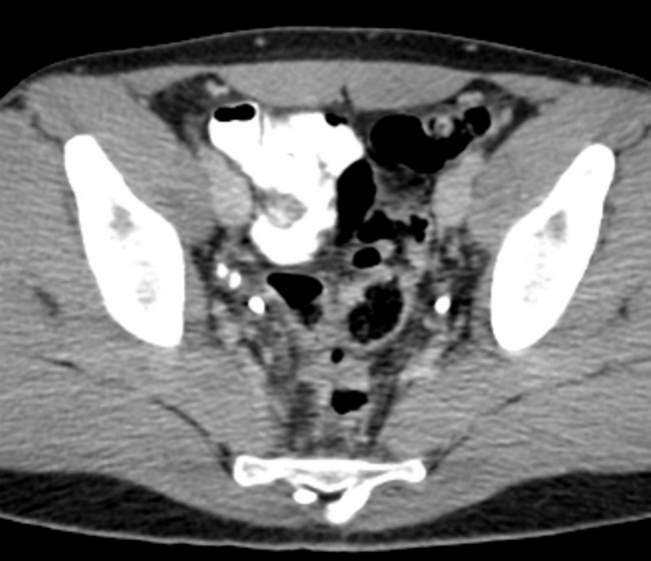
Follow-up CT scan of the pelvis, one month post-toothpick removal, shows significant improvement of sigmoid wall thickening and regression of pericolic fat stranding.
